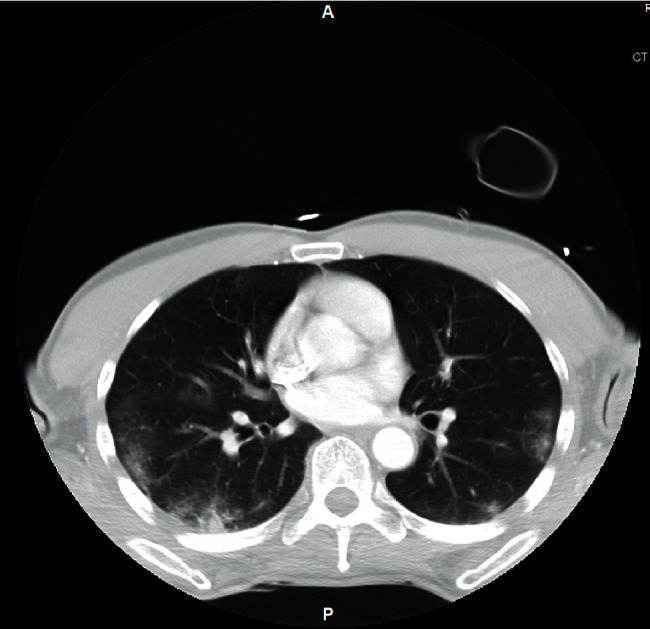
Chest CT of the COVID-19 patient showing patchy and peripheral ground glass opacities mid to lower lobe predominant (taken from another COVID-19-positive patient for comparison).
